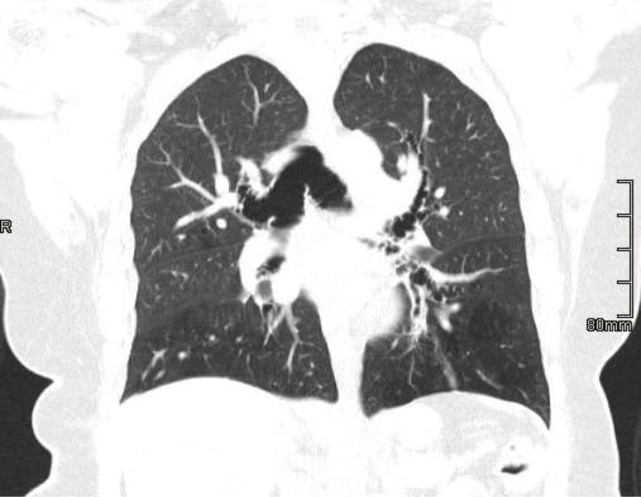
HRCT scan image highlighting bronchial dilatation (right measuring 22.5mm, left 20.4mm), varicose bronchiectasis, centrilobar, emphysema and basal fibrosis
